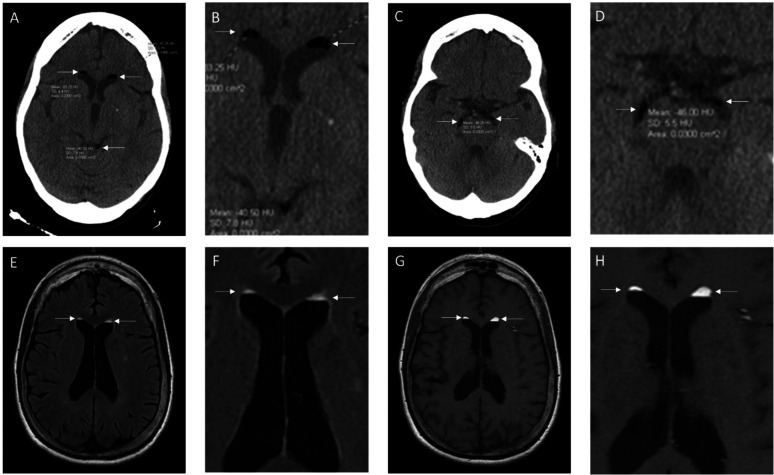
Non-contrast CT showing hypodense foci in lateral ventricles and subarachnoid space with HU (Hounsfield Units) between −40.5 and −97.25 (A,C with magnified images B,D respectively); T2 FLAIR MRI (E with magnification F) and T1 weighted MRI (G with magnification H) showing hyperintense foci in lateral ventricles overlapping foci previously seen on CT.
